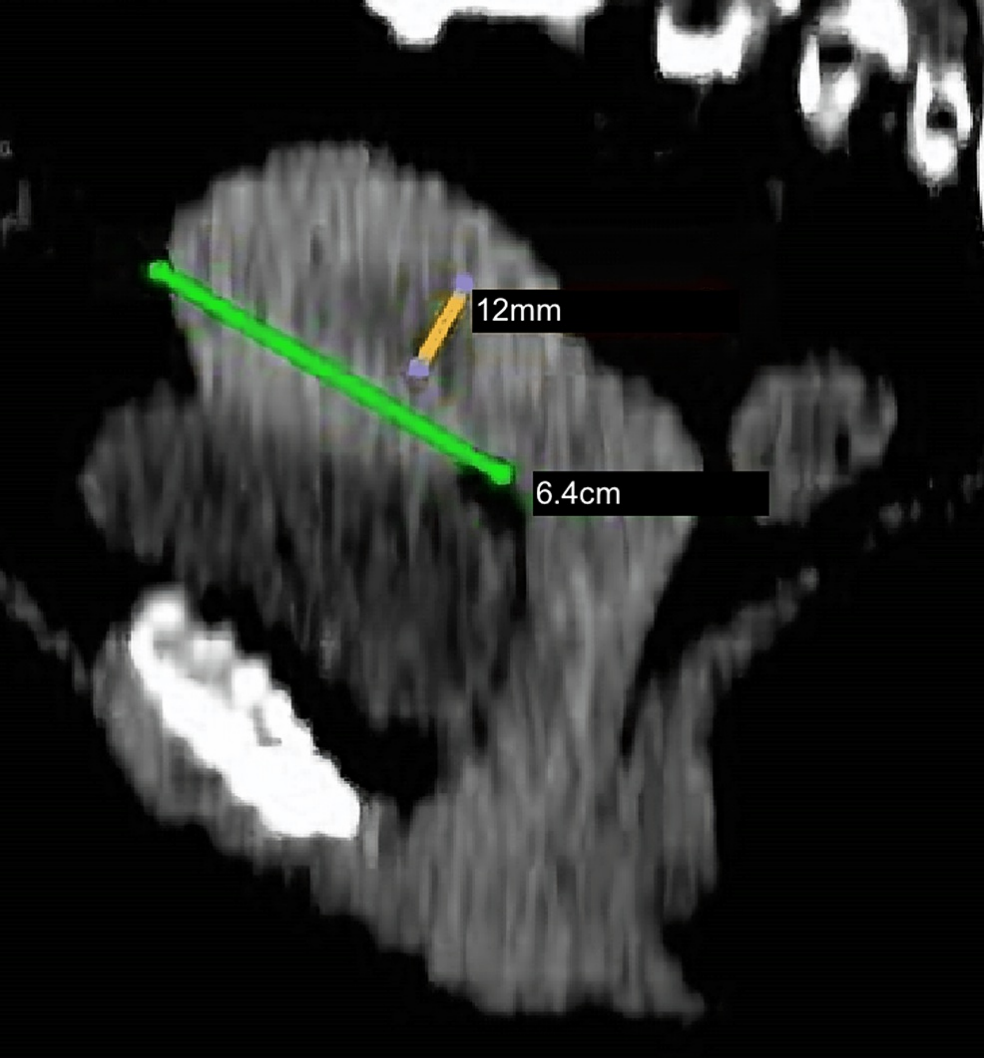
Computed tomography scan of the pelvis highlights a uterine size of 6.4cm x 5.4cm with an endometrial thickness of 12mm.
